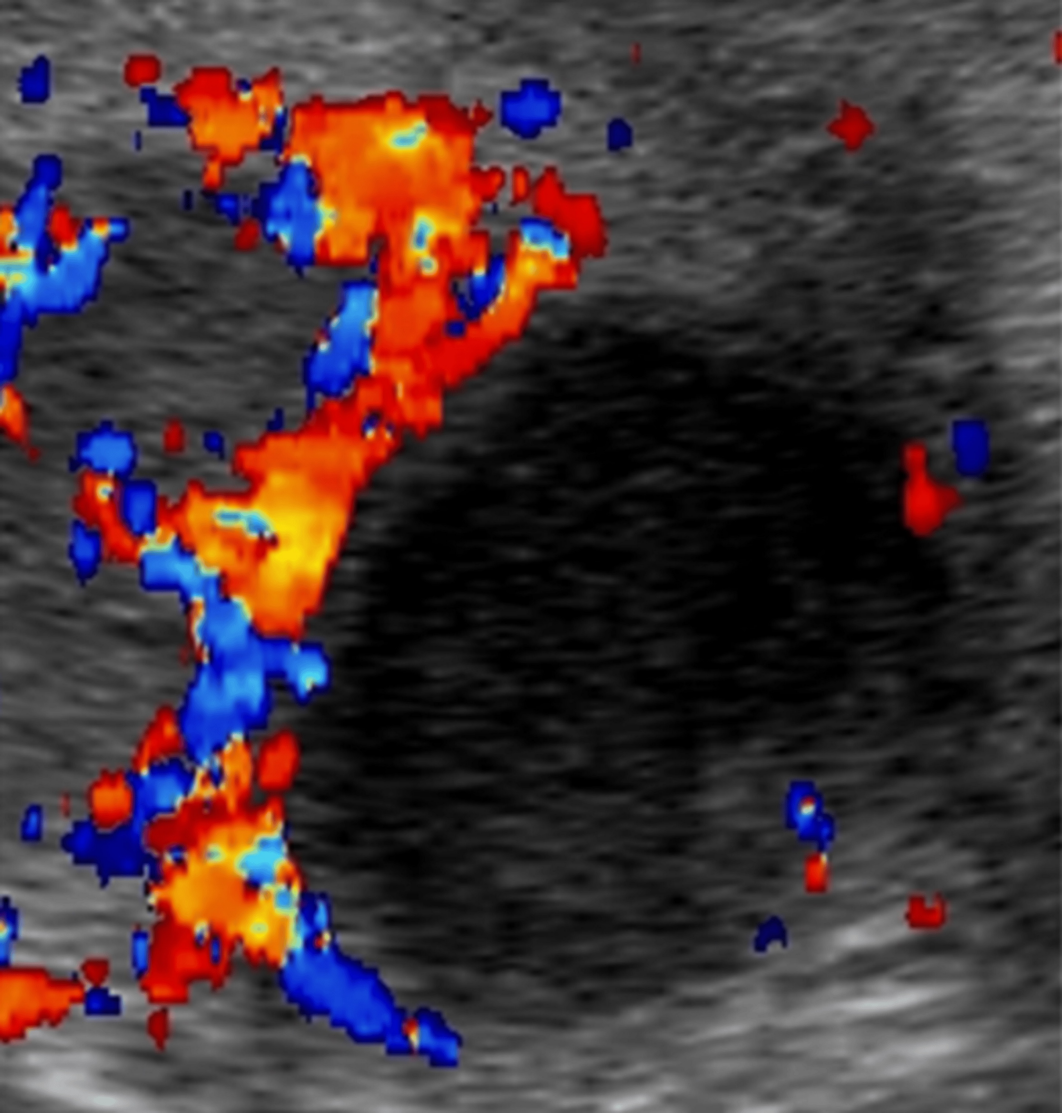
Testicular necrosis secondary to active TB infection, with increased surrounding vascular flow, as shown on Color Doppler US.
